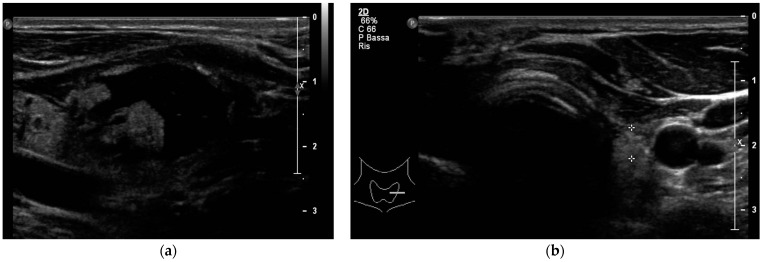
Ultrasound examination of the anterior neck region before (a) (with focus on the thyroid nodule) and after (b) thyroidectomy (with the “+” symbols delineating the size of the inhomogeneous area in left thyroid lodge attributable to post-surgical remodeling outcomes).
